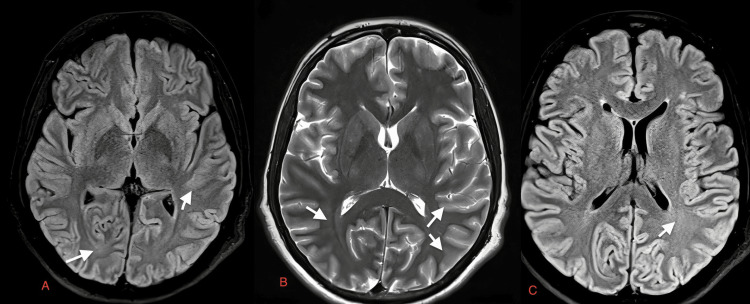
Case 5: Cortical areas of hyperintensities are observed in both parieto-occipital lobe on T2W (white arrows in B) and FLAIR images (white arrows in A and C) of a 40-year-old male patient on day one of presentation to the hospital. T2W: T2-weighted image; FLAIR: fluid-attenuated inversion recovery sequence
